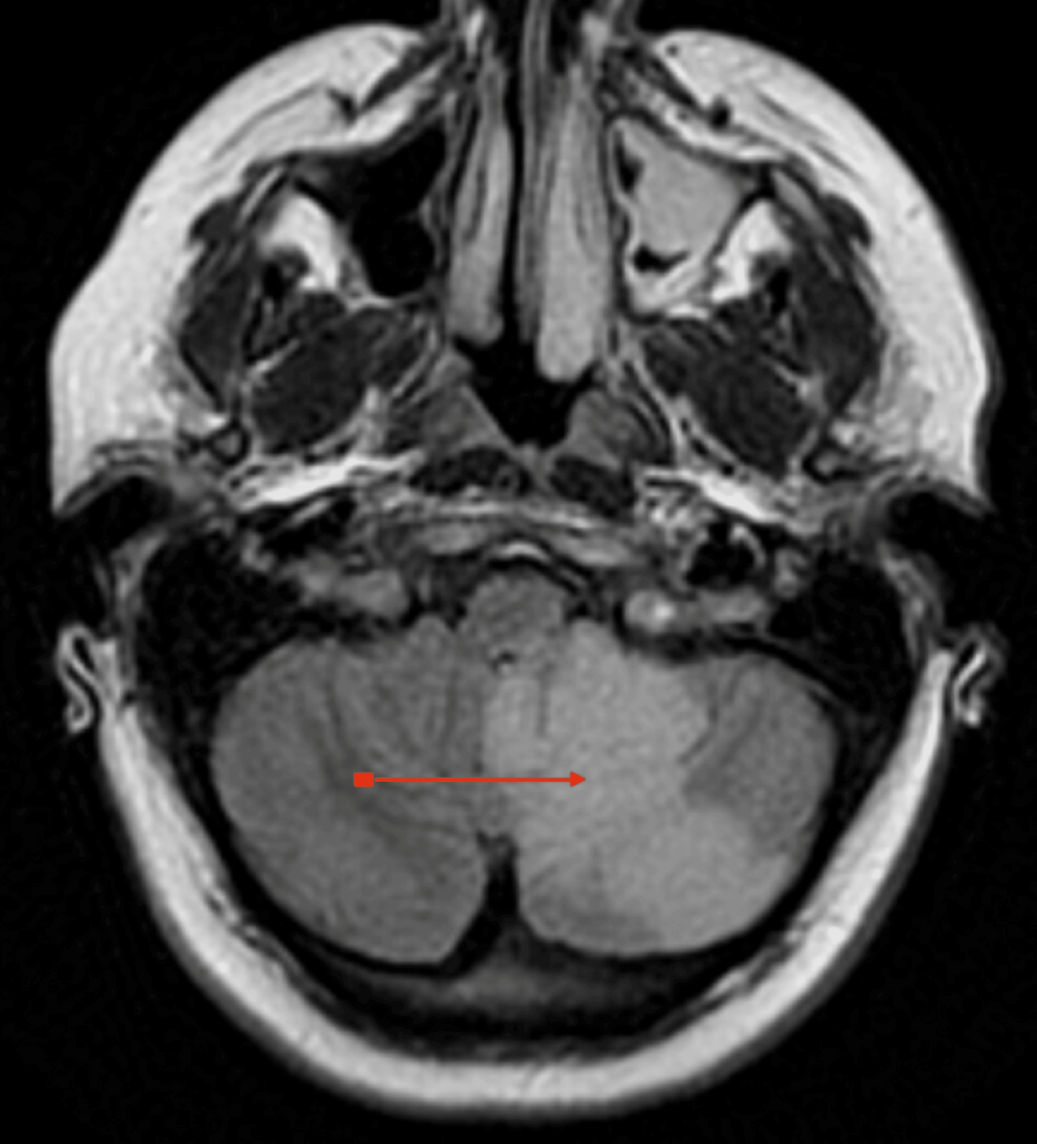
MRI of the patient's brain The red arrow depicts the area of the medulla where there is ischemia.
